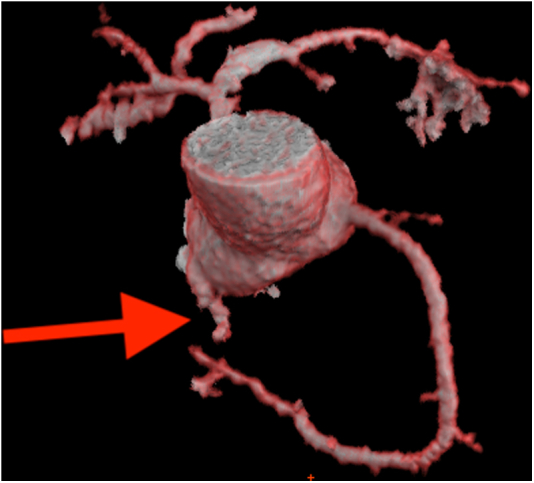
CT 3D Reconstruction CT 3D reconstruction shows a long fistulous tract connecting the noncoronary cusp to the right atrium. The fistula is pointed by the red arrow. CT = computed tomography; 3D = 3-dimensional.
